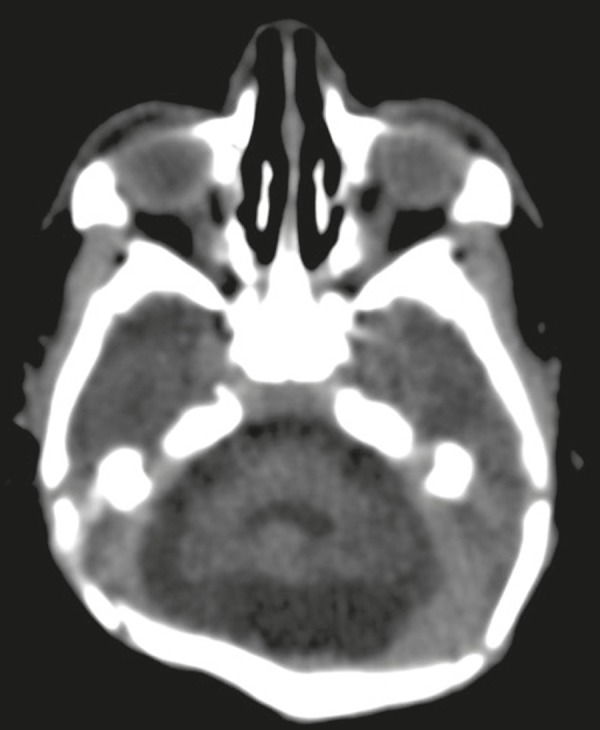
Non-contrast-enhanced CT images of the brain. Calcification patterns at
